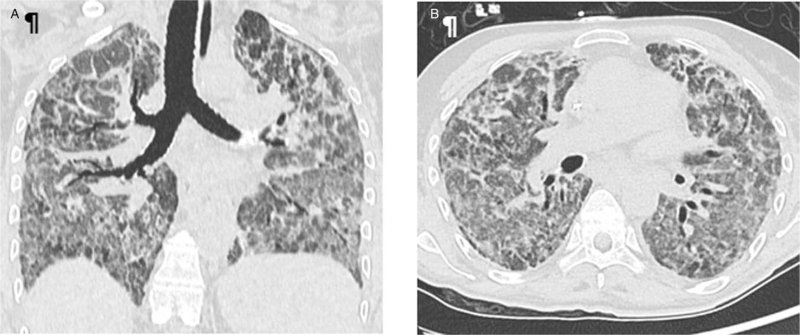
(a and b) CT lung scan: bilateral widespread ground-glass patches associated with interlobar thickenings, and banded consolidation. Note areas of parenchymal sparing, particularly at the apex.
